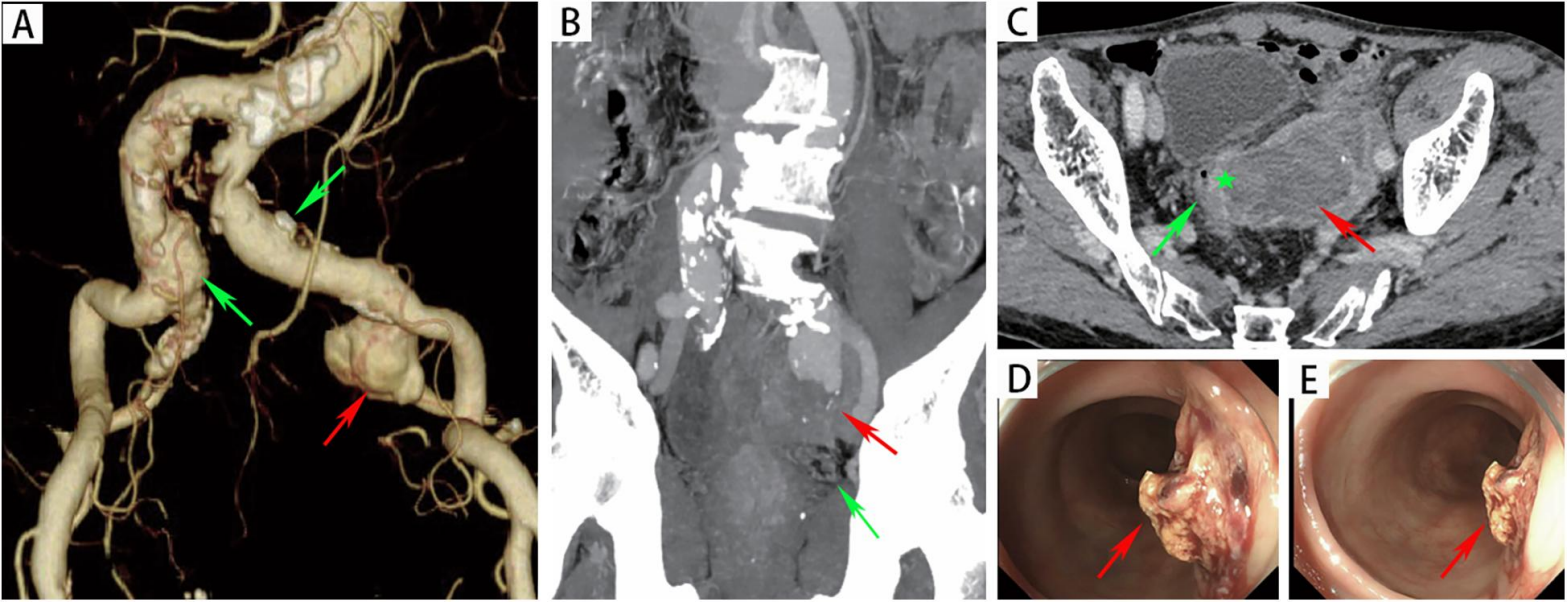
(A) CTA revealed a left internal iliac aneurysm (red arrow) and bilateral common iliac artery aneurysms (green arrow). (B) The red arrow indicated the left internal iliac artery aneurysm, with the rectum (green arrow) located below it. (C) CTA revealed that there was an unclear boundary between the left internal iliac artery aneurysm (red arrow) and the rectal wall (green pentagon). (D-E) Endoscopic evaluation revealed a raised, blood clot-adherent ulcer (arrows) on the rectal lateral wall.
